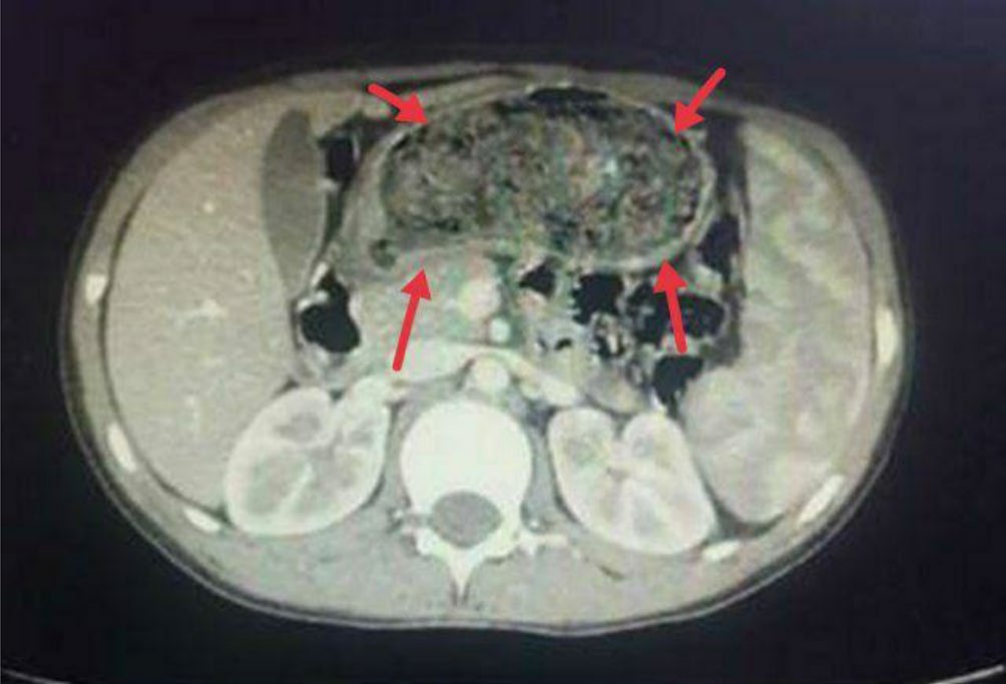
CT scan of the abdomen showing the mass of hair filling the entire stomach.
